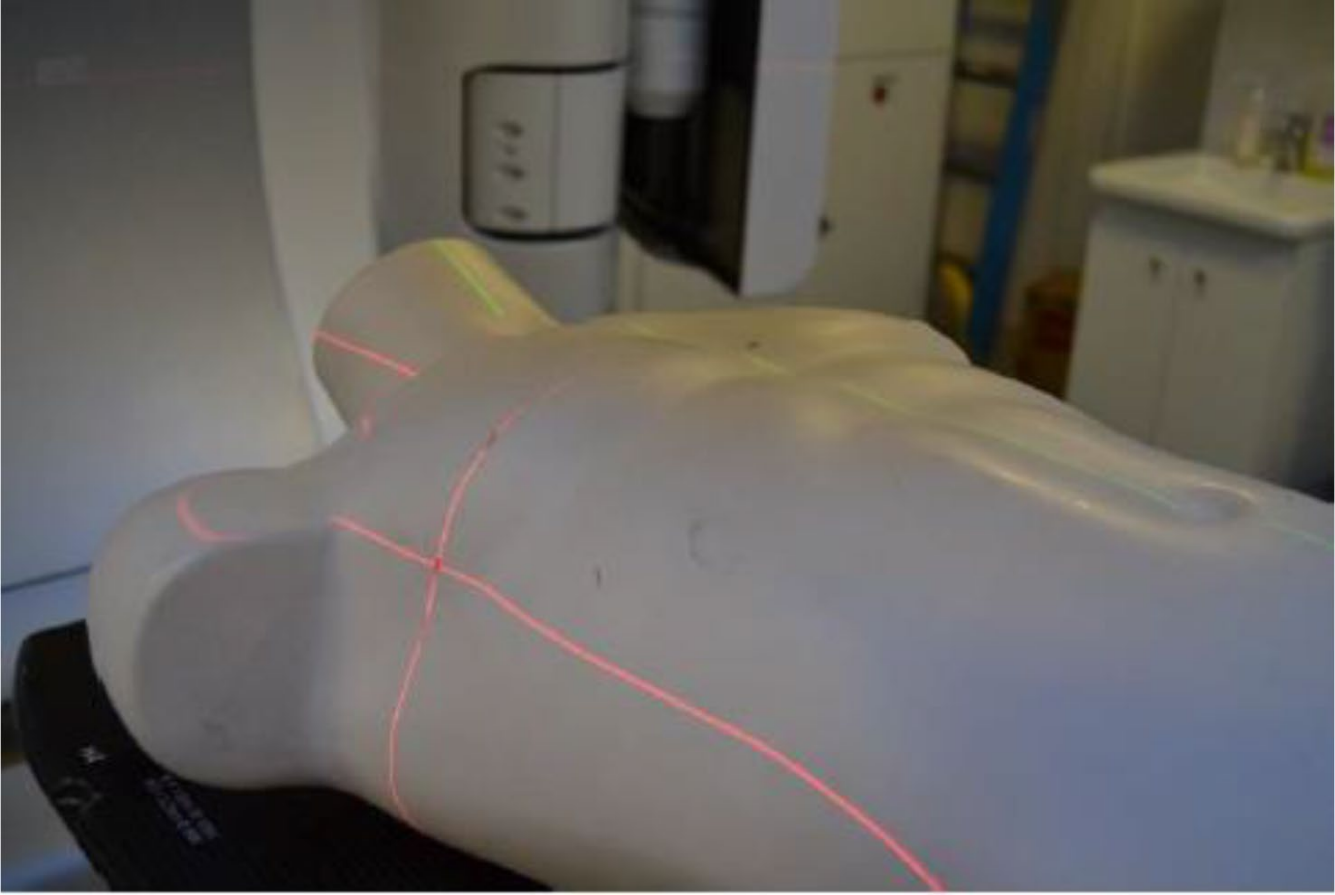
The mannequin’s placement is crucial to adjust the corresponding CT markings and ensure the the exact positioning before irradiation begins
